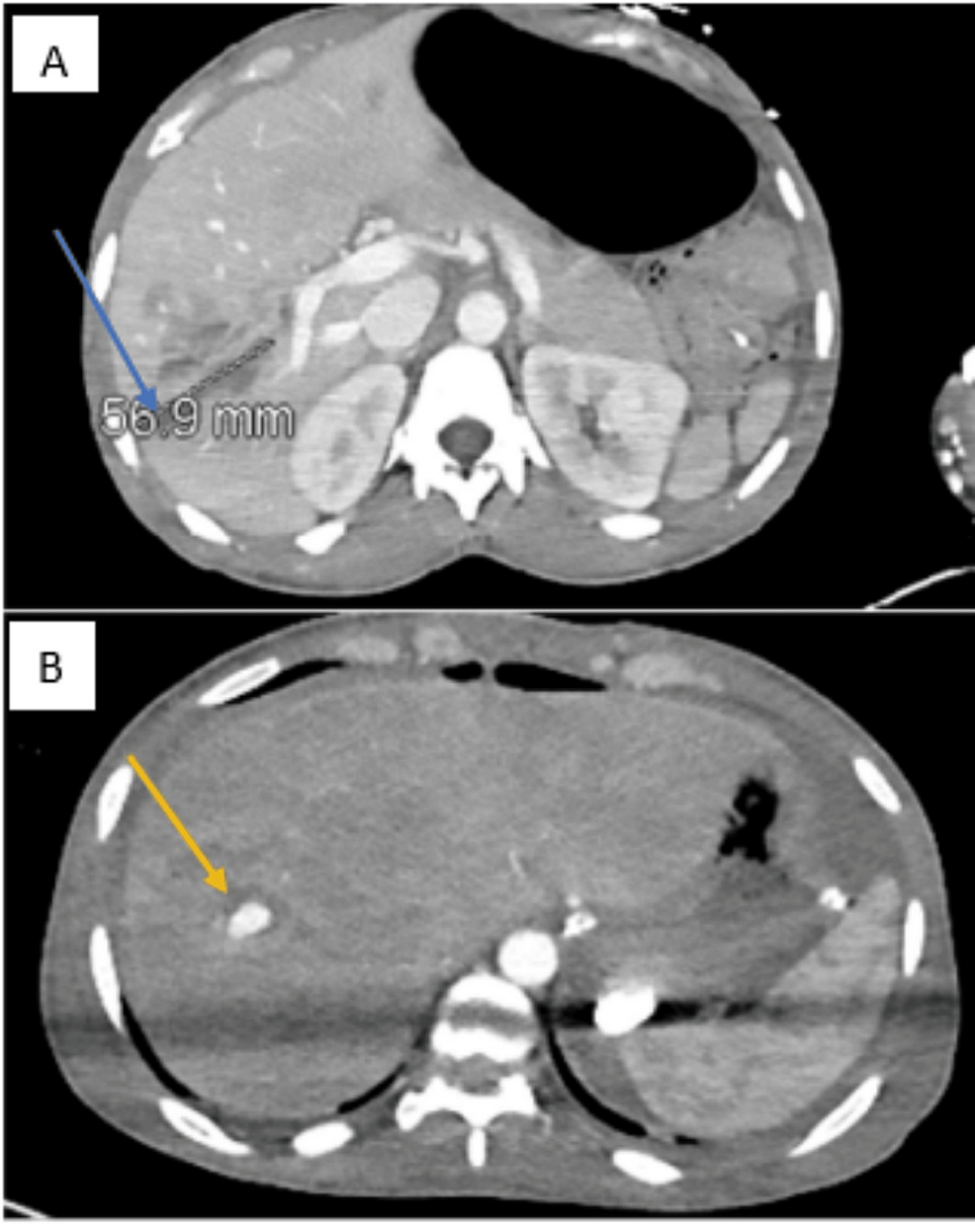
CT abdomen demonstrating hepatic artery aneurysm A- liver laceration (blue arrow); B- hepatic artery aneurysm (yellow arrow)
